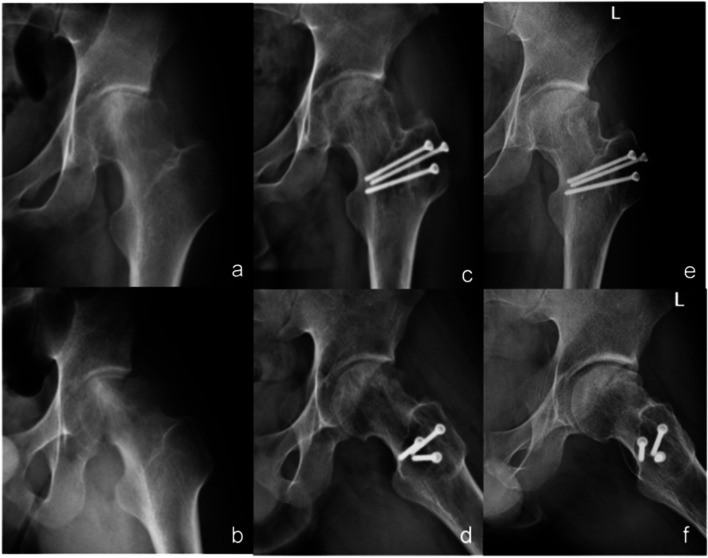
Radiographs of a 20-year-old male with idiopathic ONFH, ARCO IIIB, JIC C2. a and b A large area of bone density change can be seen on the X-rays (anterio-posterior and frog position) before the operation, and the femoral head is obviously collapsed and deformed. c and d X-rays (antero-posterior and frog position) of the patient with SHD combined with bone graft at 2 years after operation, and it can be observed that the collapse of the femoral head has not become more serious, and the bone density in the necrotic area has increased. e and f X-rays (antero-posterior and frog position) of the patient at six years after operation, it can be observed that the shape of the femoral head is maintained well, the collapse of the femoral head has not progressed, the density is increased, most of the necrotic area is repaired, the joint space remains normal, and there is no subluxation of the hip joint
